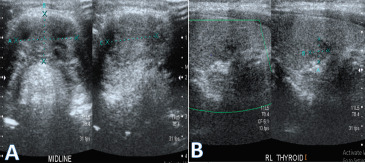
A, B. Ultrasonographic images showing thyroglossal cyst and right thyroid nodule.
